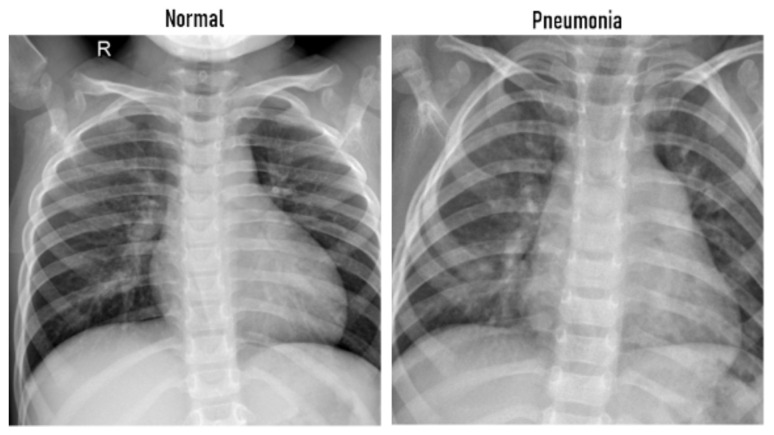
Examples of Chest X-Rays images. (Left) Normal patient, (Right) patient with pneumonia.
